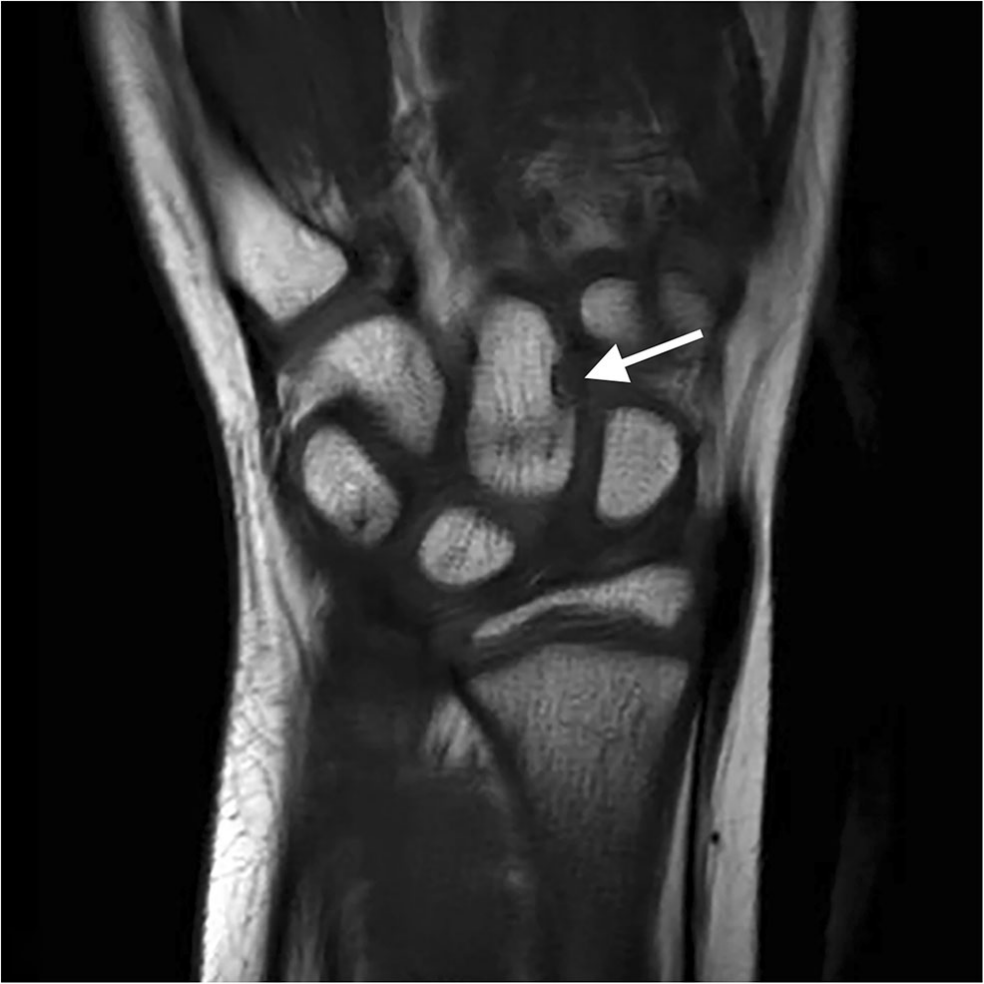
A 10-year-old healthy girl with a coronal T1-weighted image showing a bony depression on the radial side of the capitate (arrow). This is a normal depression that can be seen in the carpal bones of growing children and should not be mistaken for pathology (erosive disease)
